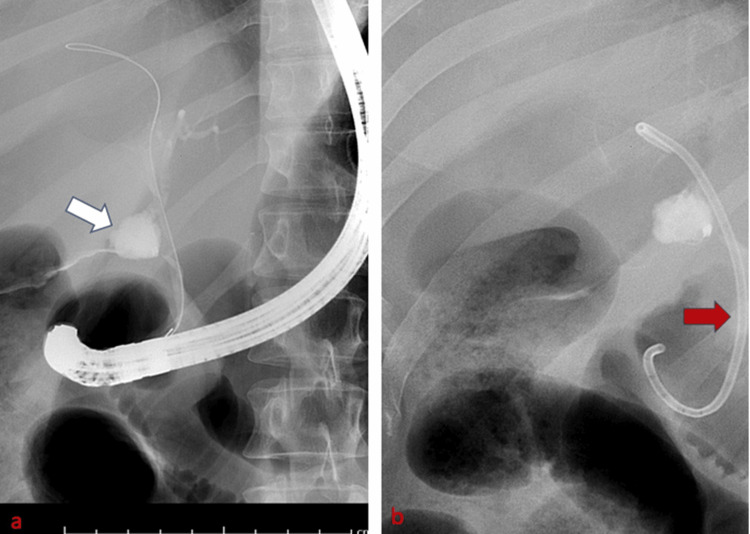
Selected images of endoscopic retrograde cholangiopancreatography Intraoperative radiographic images of endoscopic retrograde cholangiopancreatography (ERCP) demonstrating contrast leak (white arrow), after injecting contrast through the ampulla of Vater (a), concerning bile duct injury, followed by stent placement (red arrow) (b).
